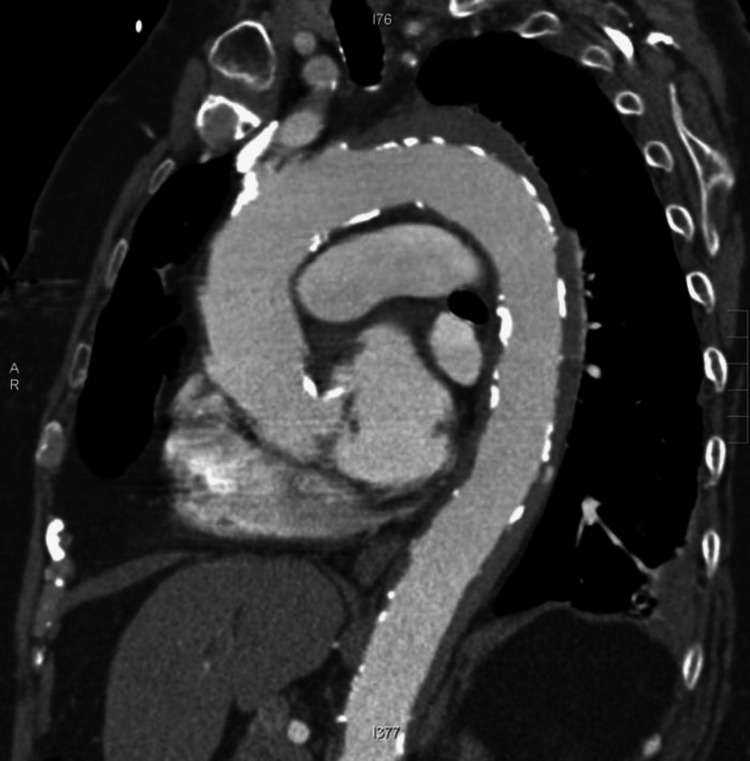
CT axial showing aortic intramural hematoma
